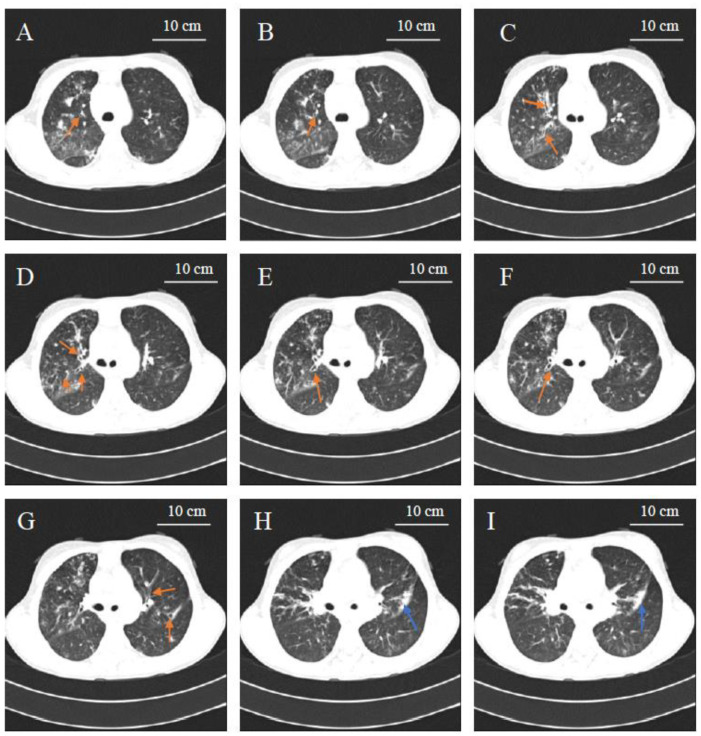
Axial computed tomography (CT) image demonstrating multiple bronchiectasis in the middle lobe of the right lung field (indicated in the A–F panels with orange arrows), left lower lobe bronchiectasis (highlighted in panel G with orange arrows), pulmonary sclerosis in the lower lobe of left lung field (indicated in panels H and I with blue arrow). CT instrument: Siemens Somatom Sensation 16 EDE 5,6 mSv.
